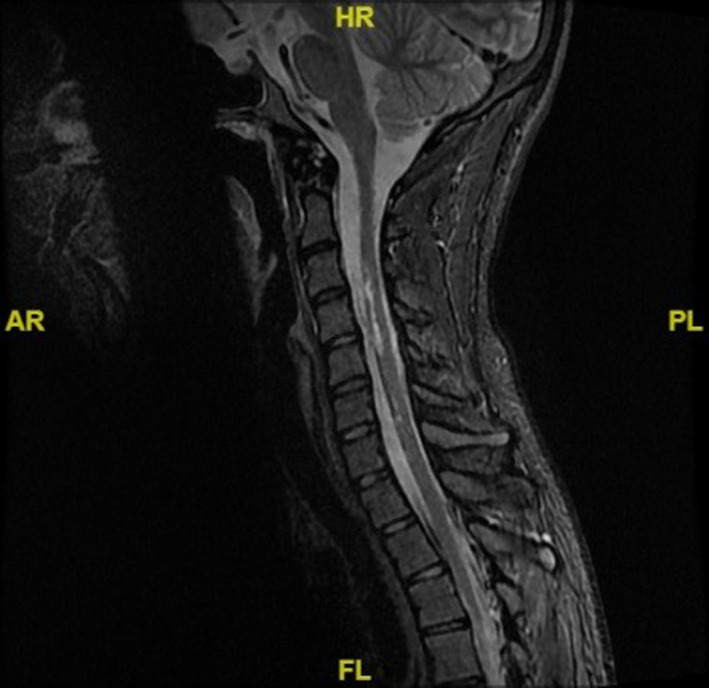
MRI Sagittal T‐2 image of cervical spine showing multilevel (C 2‐ C6) spinal contusion without evidence of spinal fracture or instability
